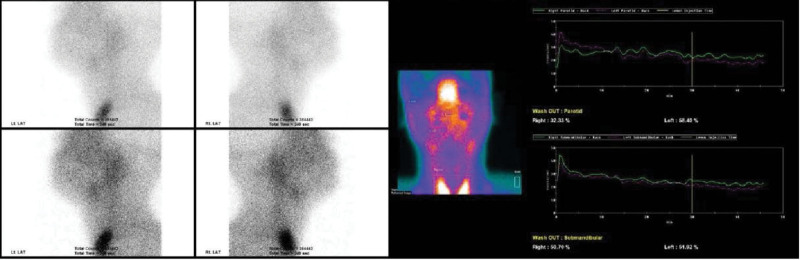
99mTc scintigraphy image. Accumulation in the bilateral parotid and submandibular glands is extremely low, and almost no accumulation is observed. In addition, almost no secretion by lemon juice stimulation is observed, suggesting severe functional decline. On the other hand, thyroid accumulation is normal.
